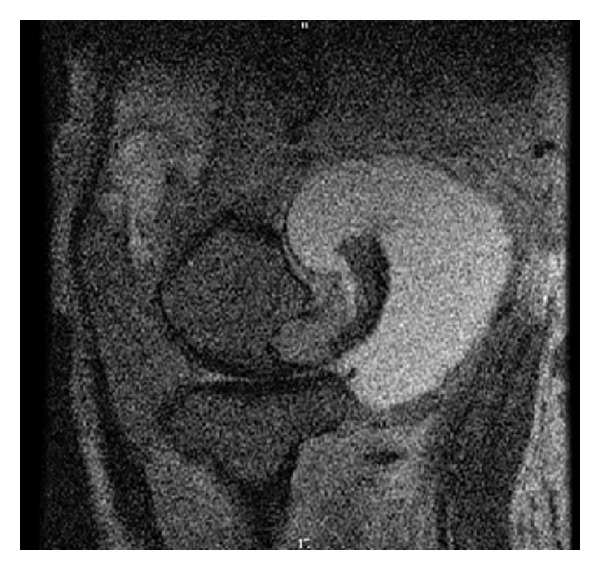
Suprapatellar and posterior knee effusions are visualized with MRI.
